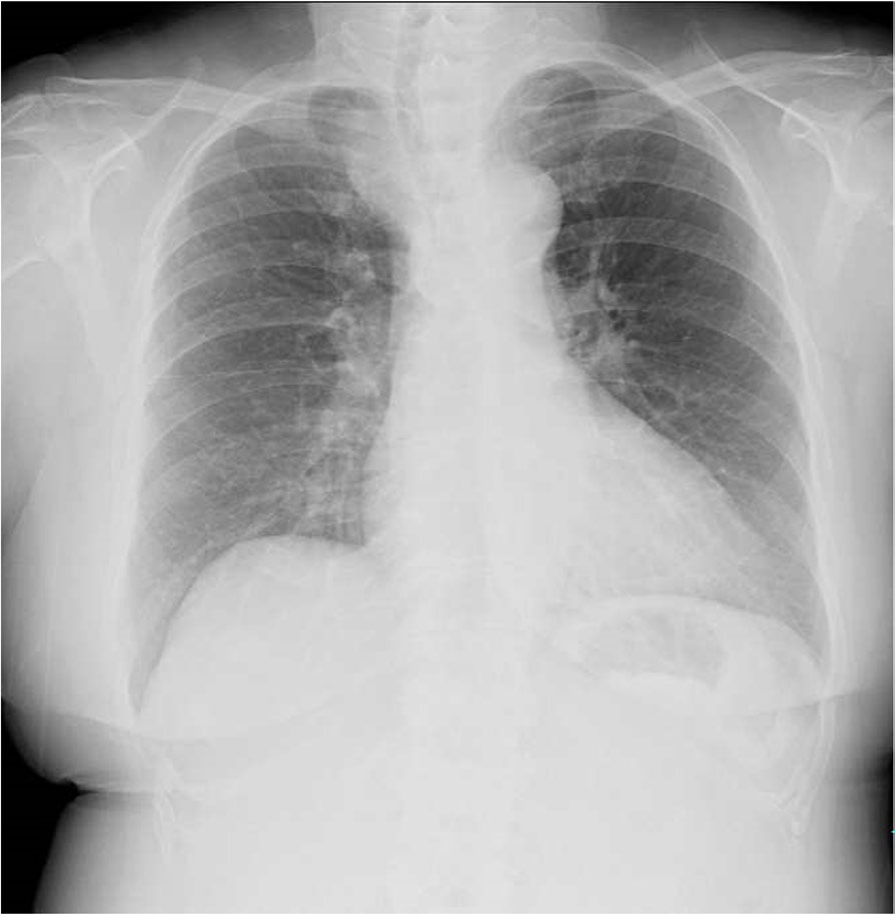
Postoperative chest X-ray findings. The tracheal stenosis had improved at 1 month after the surgery
